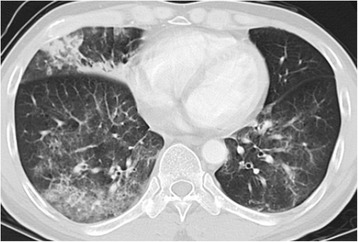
Computed tomography images of nivolumab-induced pneumonitis in Case 4. Multiple consolidations and ground-glass opacities developed at both lungs
